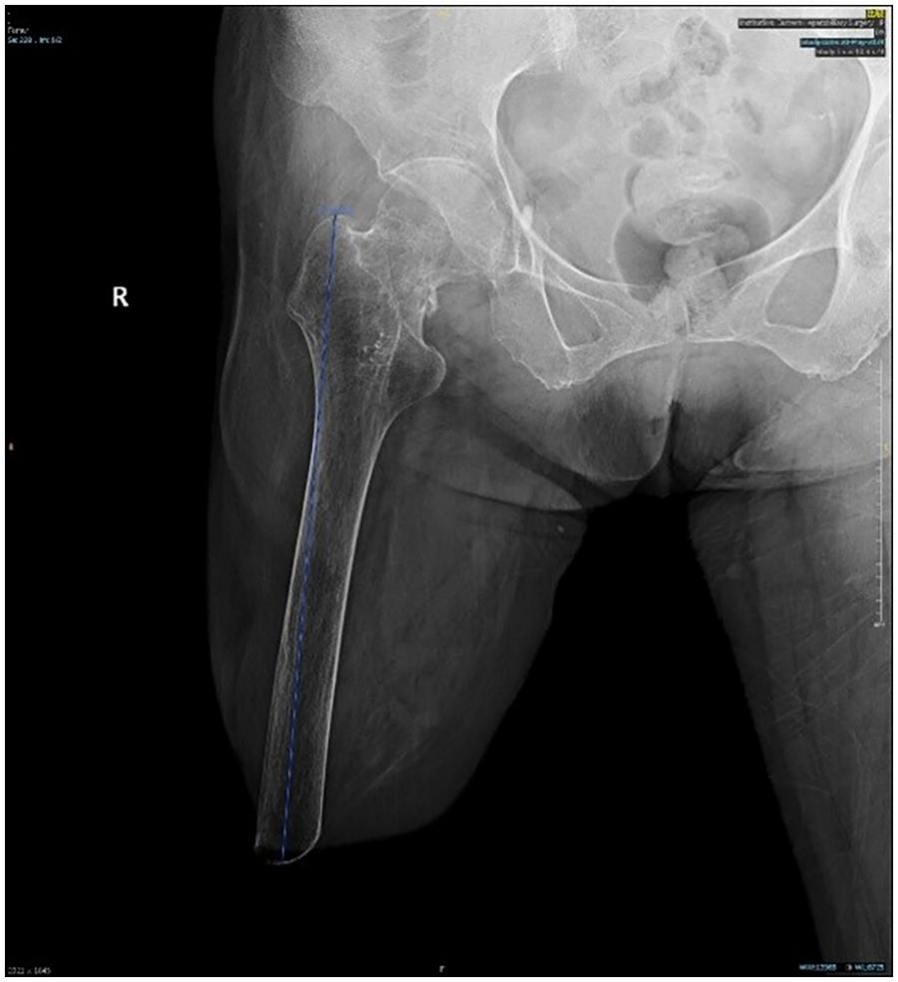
Preoperative antero-posterior radiograph of the pelvis showing a displaced femoral neck fracture.
